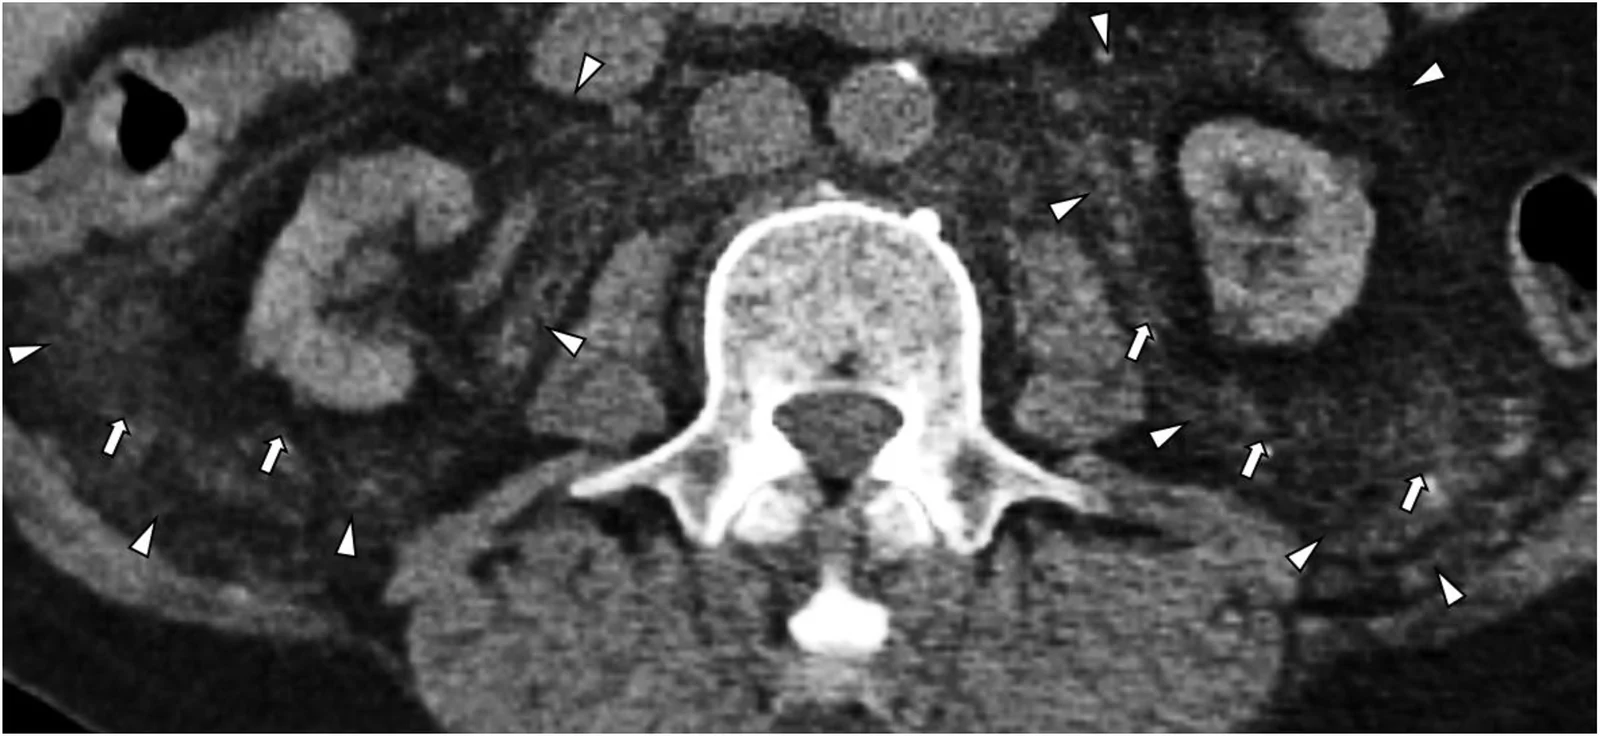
Axial noncontrast CT of the abdomen demonstrating bilaterally atrophic kidneys and diffuse amorphous hyperattenuating haziness and ill-defined nodularity throughout the bilateral retroperitoneal perinephric compartments (white arrowheads) with subtle areas of punctate calcification (white arrows) and relative sparing of the immediate perinephric fat.
